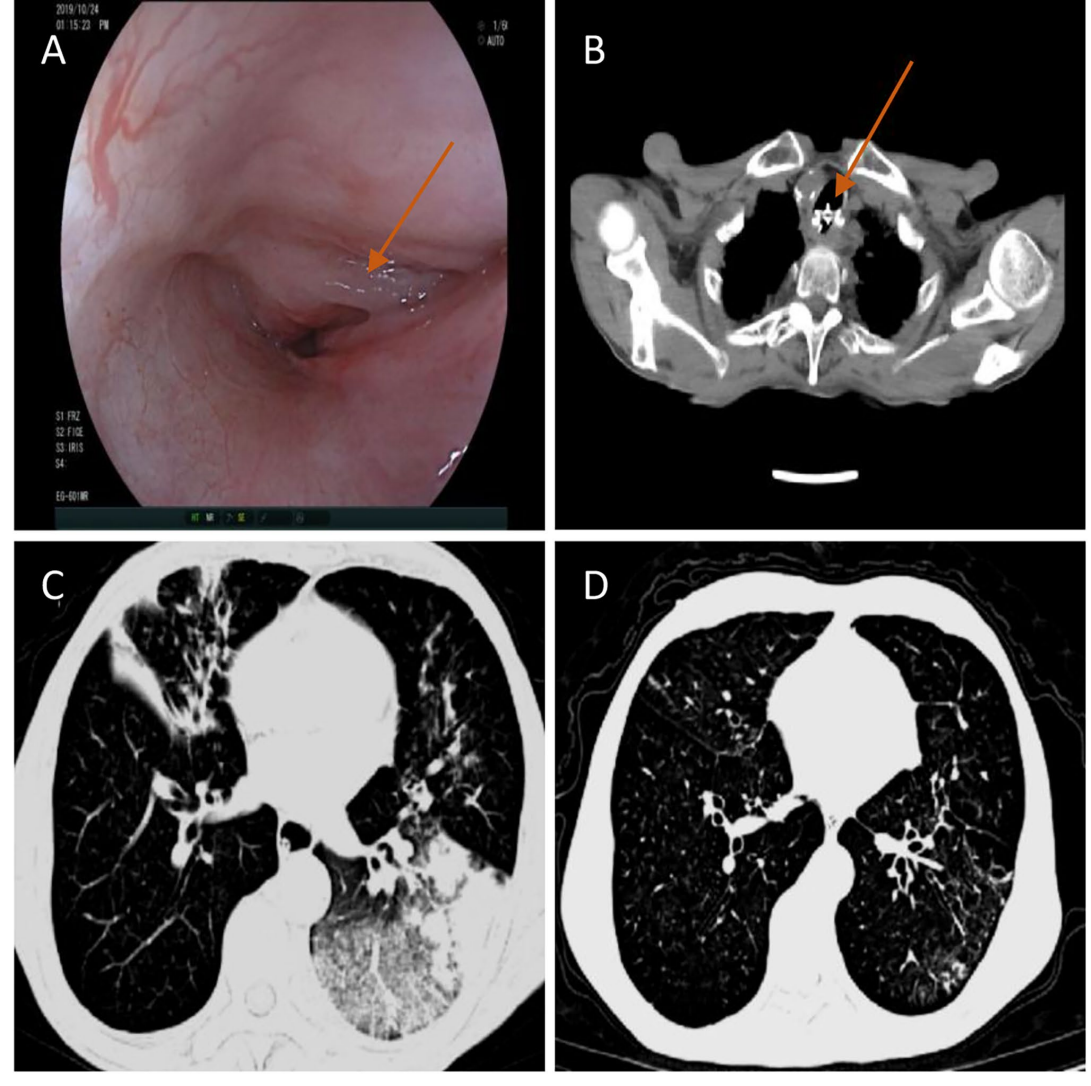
Follow-up evaluation 90 days after TEF occlusion in patients with esophageal cancer. (A) Gastroscopy examination showed epithelialization on the surface of the occluder, good adhesion to the esophageal wall, and no residual fistula; (B) After 90 days of TEF occlusion, chest CT was performed to confirm the position of the occluder. (C and D) Comparison of pre- and postoperative chest CT showed a significant improvement in pulmonary infection after TEF occlusion. The location of the occlusion device is indicated by the arrow
